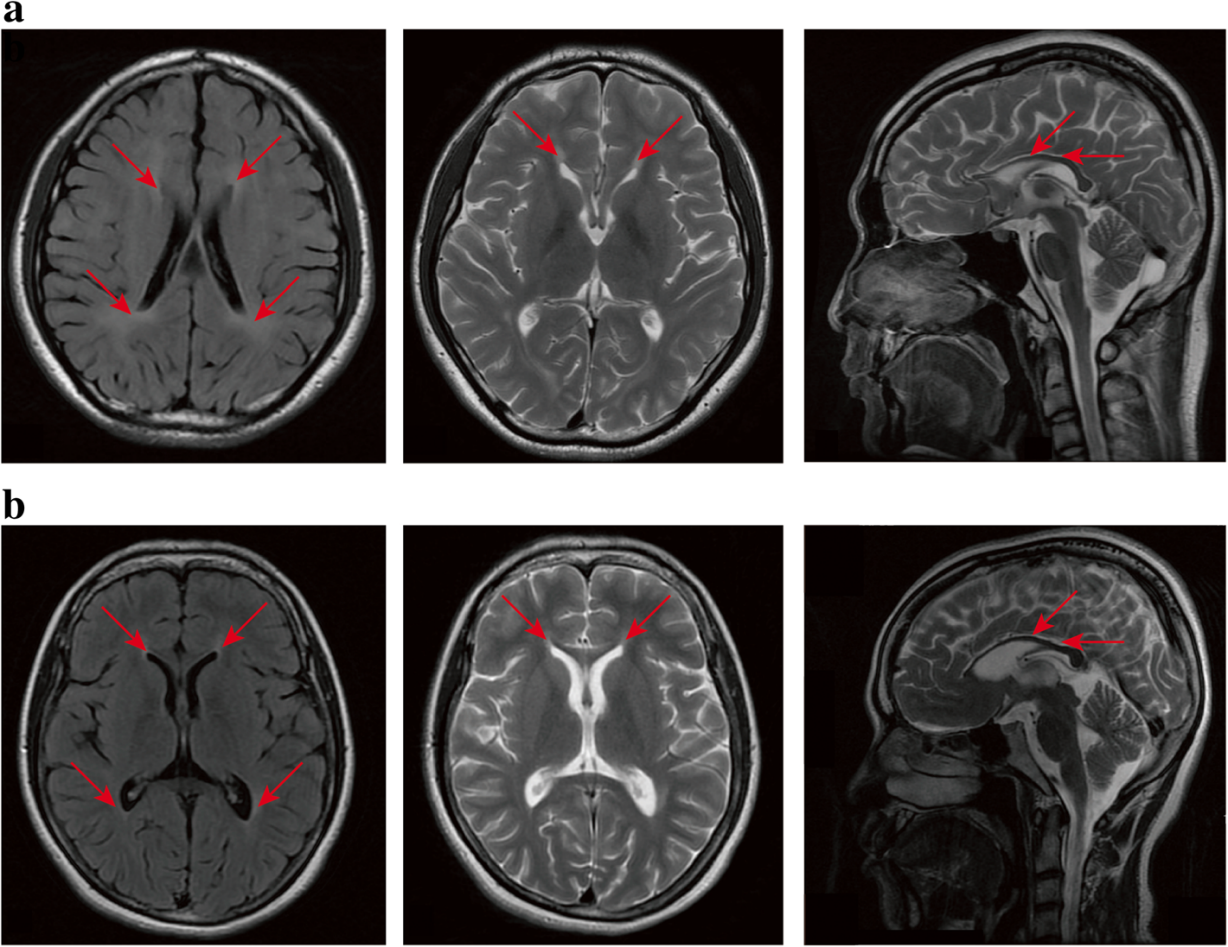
Brain MRI of case 1 and case 2. An ‘ears of the lynx’ appearance and thinning of corpus callosum were seen in case 1 (a) and case 2 (b)
